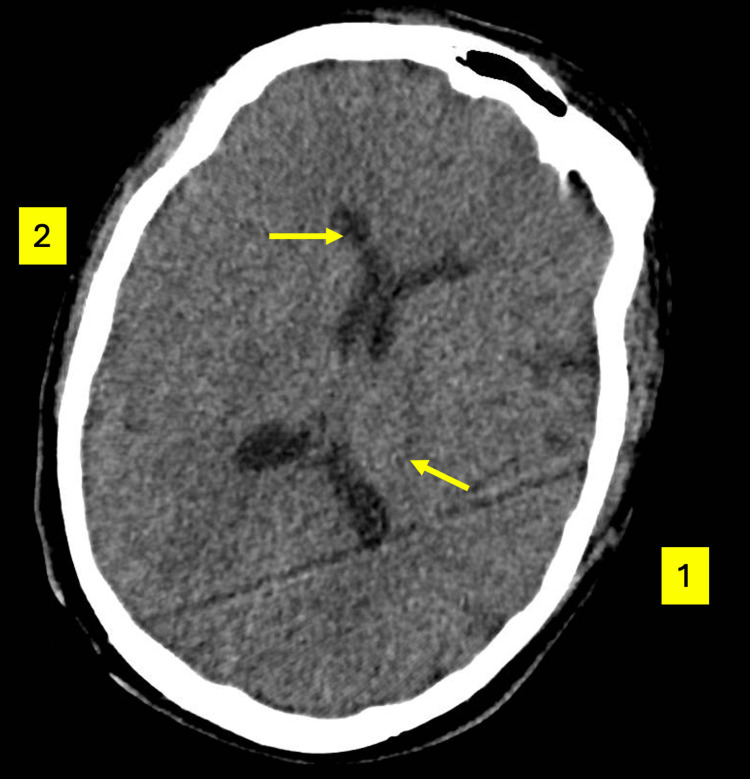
Initial non-contrast axial computed tomography (CT) of the head Axial view at the level of the thalamus is shown. No acute intracranial hemorrhage, midline shift, or large-vessel territory infarction is present. Arrow 1 indicates the right thalamus, and Arrow 2 points to the anterior horn of the right lateral ventricle, both demonstrating normal morphology and attenuation. A diagonal linear streak across the posterior right hemisphere is a motion artifact. Normal pneumatization of the frontal sinus is visible at the anterior aspect of the skull. The absence of structural pathology was critical in excluding primary neurological causes for the patient’s altered sensorium and muscular rigidity, redirecting focus toward a metabolic etiology.
